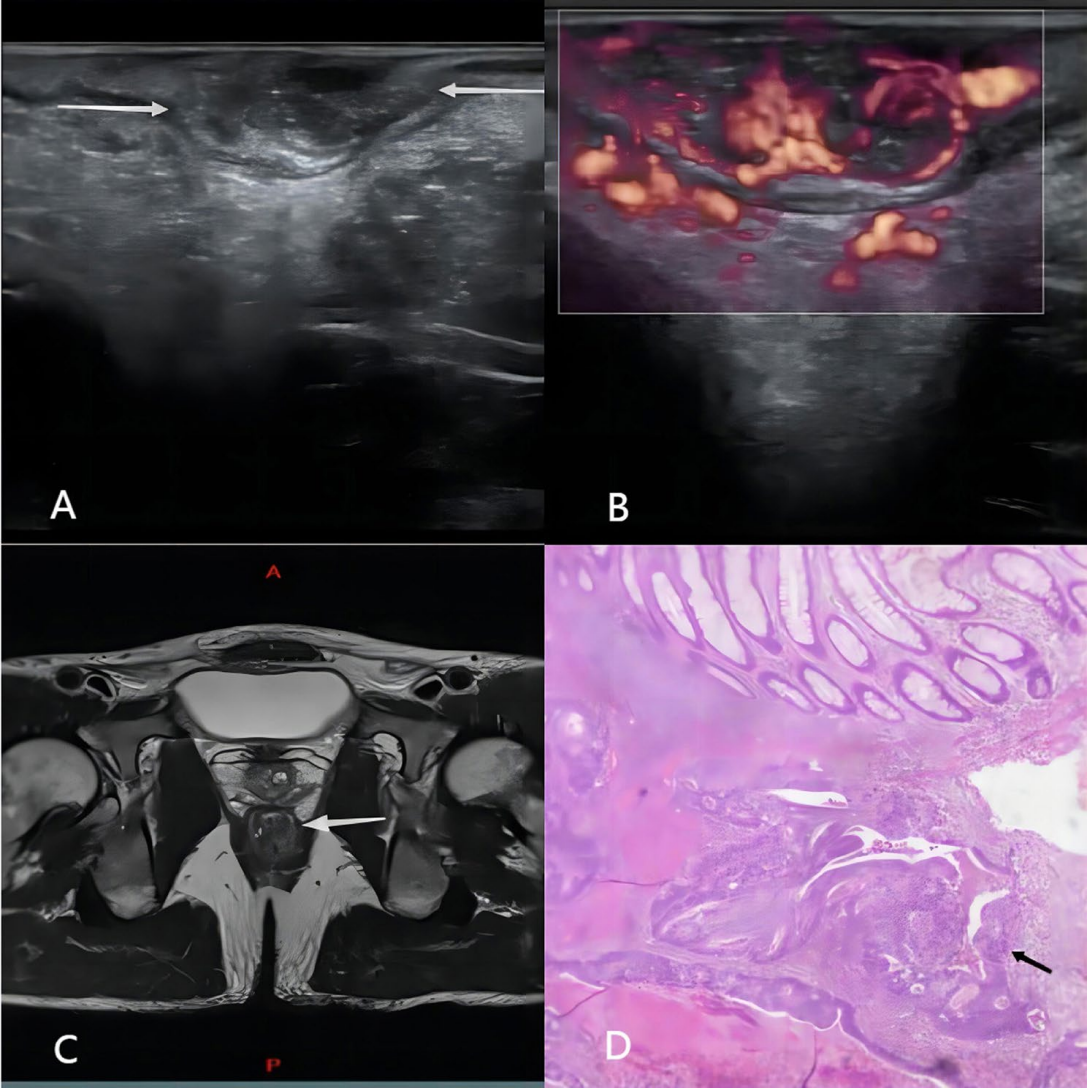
Images of a 47-year-old man with a T1 stage rectal tumor. (A) Two-dimensional endorectal biplane ultrasound of the line array revealed a hypoechoic mass on the rectal wall that was confined to the submucosa with a continuous hyper-echoic submucosa (arrow); the diagnosis was T1 rectal cancer. (B) An MFI mode image revealed that the microblood flow signals of the mass were abundant; there were more than four blood flow streams and spread to more than 50% of the maximum section of the tumor. (C) Magnetic resonance imaging revealing thickening of the wall of the lower rectum under peritoneal reflection (arrow); this was diagnosed as T1 rectal cancer. (D) The pathological diagnosis was moderately differentiated adenocarcinoma stage T1 as ulcerative type, and the cancerous tissue adjacent to the submucosa did not exceed the submucosa (arrow)
